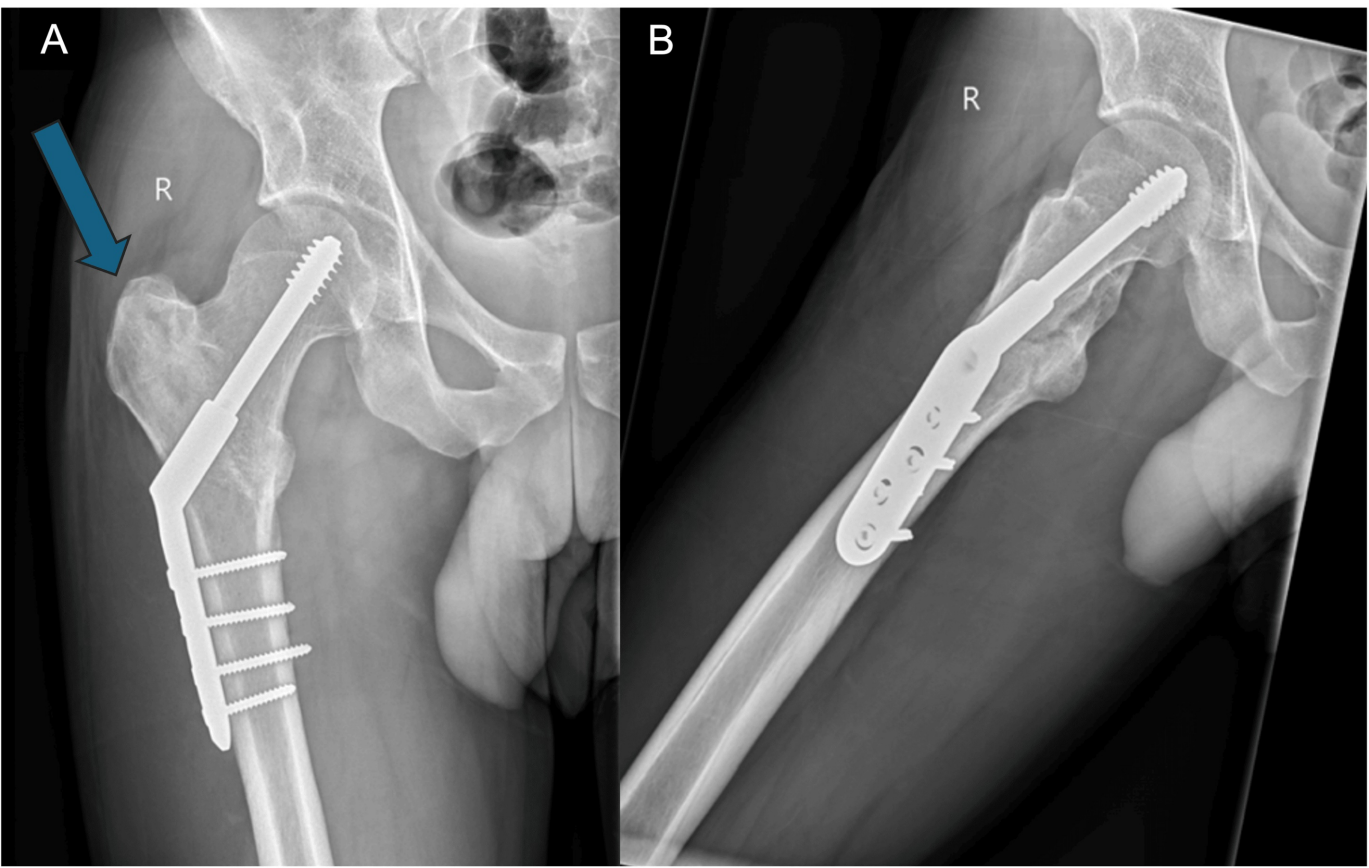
Postoperative radiographs of the right hip at six weeks (A) Anteroposterior view and (B) lateral view demonstrating position of dynamic hip screw and union of fracture (arrow)
